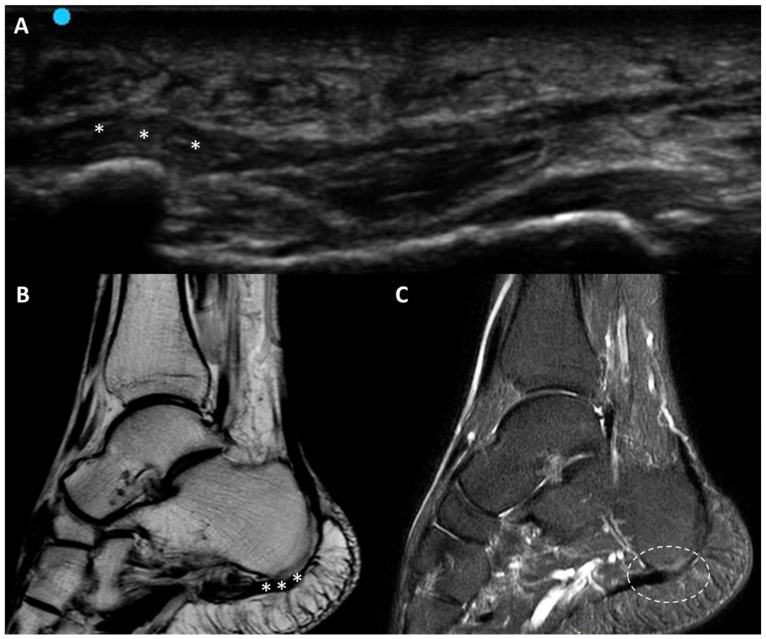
Plantar fasciitis. *: regions of increased plantar fascia thickness. (A) US examination demonstrating focal thickening and hypoechogenicity of the fascia. (B) Magnetic resonance imaging (MRI) using a dual proton–density turbo spin echo (DP_TSE) sequence, highlighting focal thickening. (C) MRI with a short tai inversion recovery (STIR) sequence, showing perilesional edema and signal intensity alterations consistent with inflammation.
